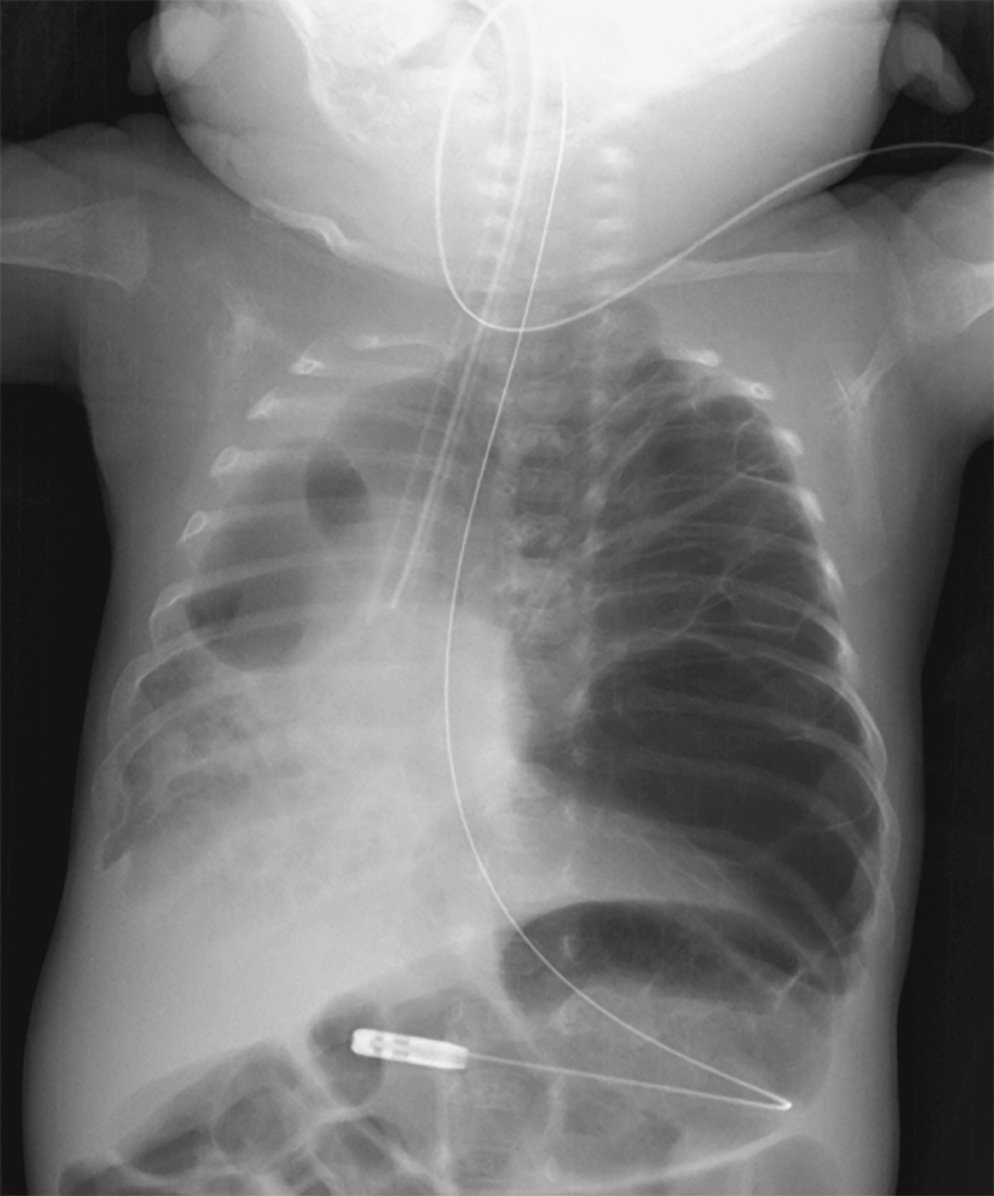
Chest radiograph showing selective right main bronchial intubation for left-sided pulmonary interstitial emphysema (PIE). The endotracheal tube tip is positioned in the right main bronchus to minimize ventilation to the left lung. The left lung shows severe emphysematous changes with multiple large cystic lesions, causing significant rightward mediastinal shift.
